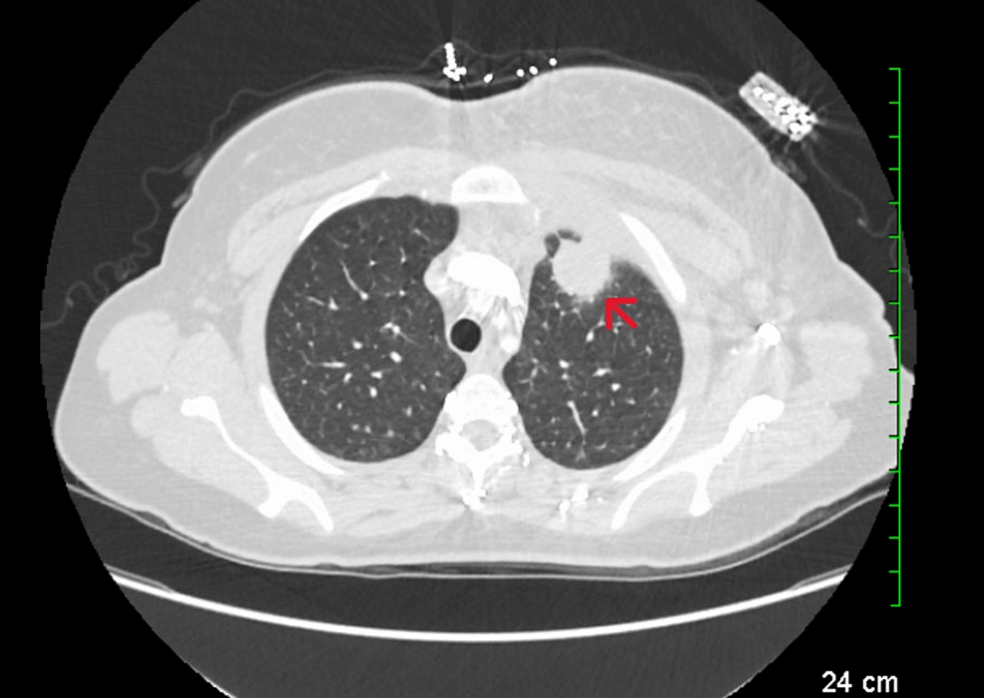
Initial CTA chest CTA: Computed tomography angiography The red arrow shows the sub-apical, mass-like lesion (26.8 mm x 26.5 mm)
